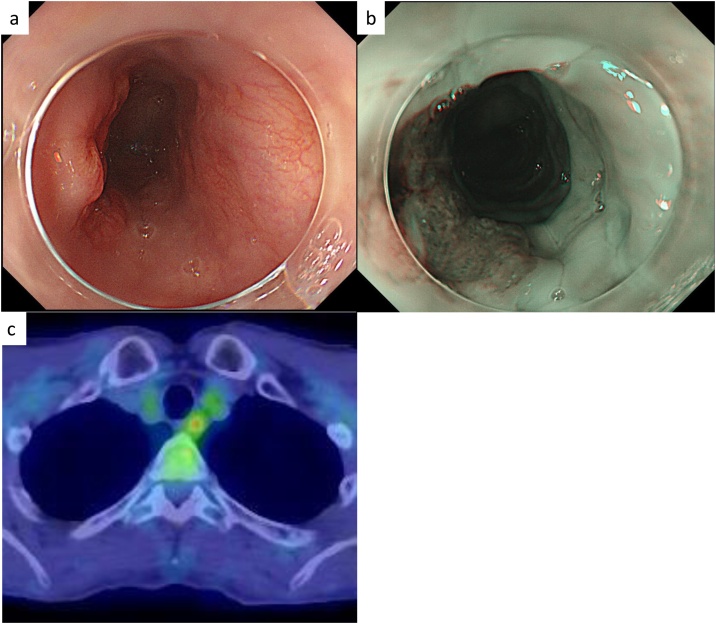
Examination before chemoradiotherapy. a: Upper gastrointestinal endoscopy showing a type 0-IIa + IIc elevated lesion located 22–23 cm from the incisors. b: Narrow-band imaging showing a brownish area at the elevated lesion. c: 18F-deoxyglucose (FDG) positron emission tomography showing high FDG uptake in the thickened wall.
